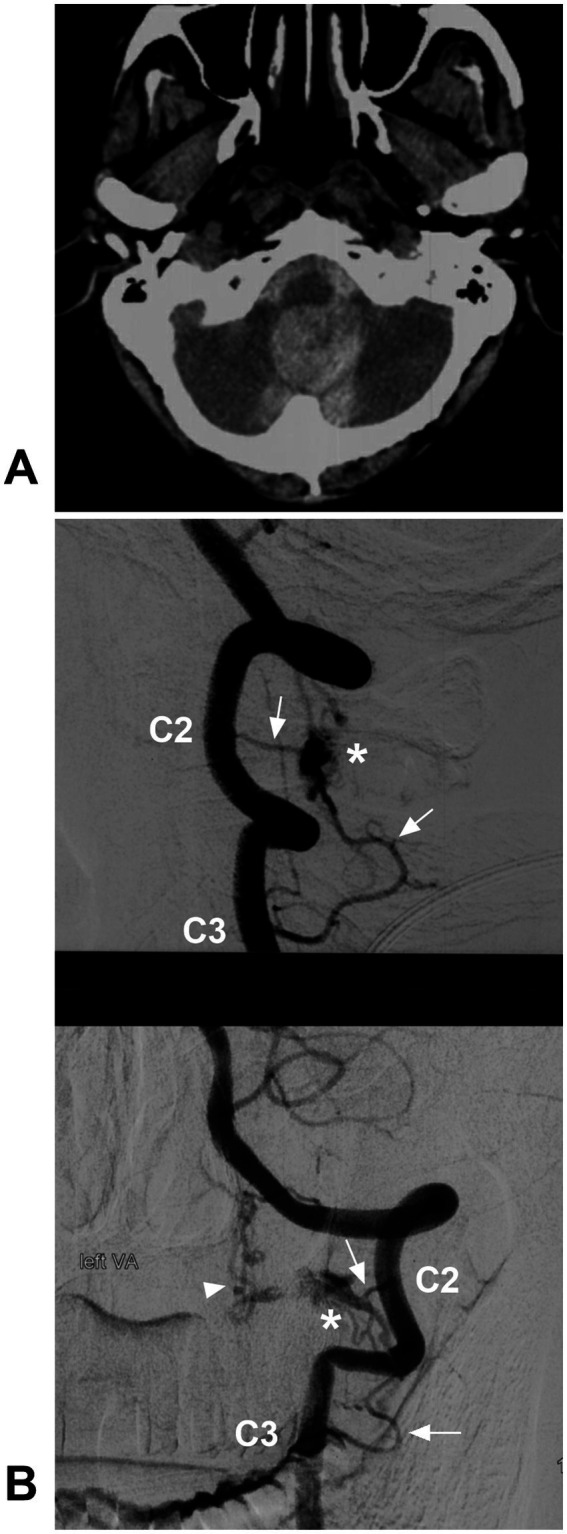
Ruptured CCJ RAVF with upward drainage. (A) CT showing subarachnoid and cisterna magna hemorrhage. (B) Lateral view (upper panel) and anterior posterior view (below panel) DSA of the VA showing an RAVF (asterisks) fed by the C2 and C3 radicular arteries (arrows) and with upward venous drainage (arrowhead in below panel). AVF, arteriovenous fistula; C2 and C3, second and third cervical vertebrae; CCJ, craniocervical junction; CT, computed tomography; DSA, digital subtracted angiography; RAVF, radicular AVF; VA, vertebral artery.
